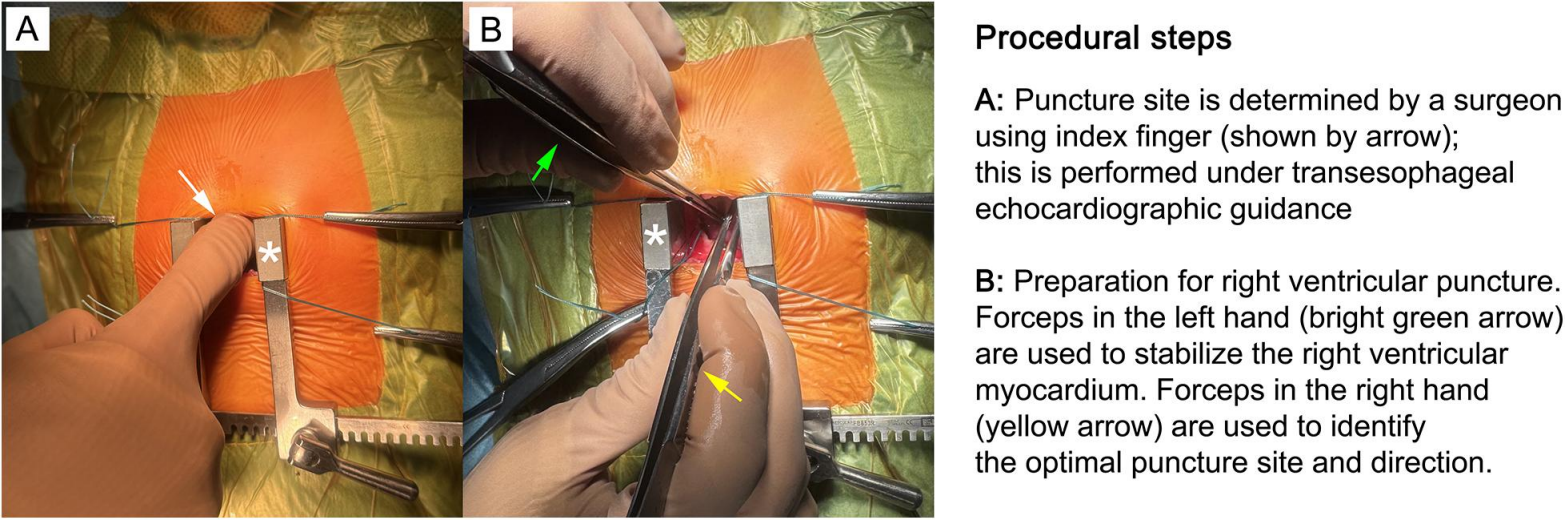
Methods for determining the correct location for right ventricular (RV) puncture. (A) Using the right index finger (shown by arrow), a surgeon identifies the approximate puncture site under transesophageal echocardiographic (TEE) guidance. (B) With the left hand, the surgeon uses forceps (bright green arrow) to stabilize the RV myocardium; forceps in the right hand (yellow arrow) are used to identify the optimal puncture site and direction. The surgeon can apply some pressure with tweezers at the point of the intended puncture of the RV. The trajectory of the guide and the angle at which the guide will approach the VSD are visible on the echocardiogram monitor. All these manipulations allow choosing the optimal puncture site and, thus, achieving the best result, avoiding repeated puncture or mechanical injury to the wall of the interventricular septum during the passage of the guide into the defect. The retractor is shown by asterisk on both panels. The images are taken in our own surgery department, hybrid room, 11-month old patient with perimembranous ventricular septal defect.
